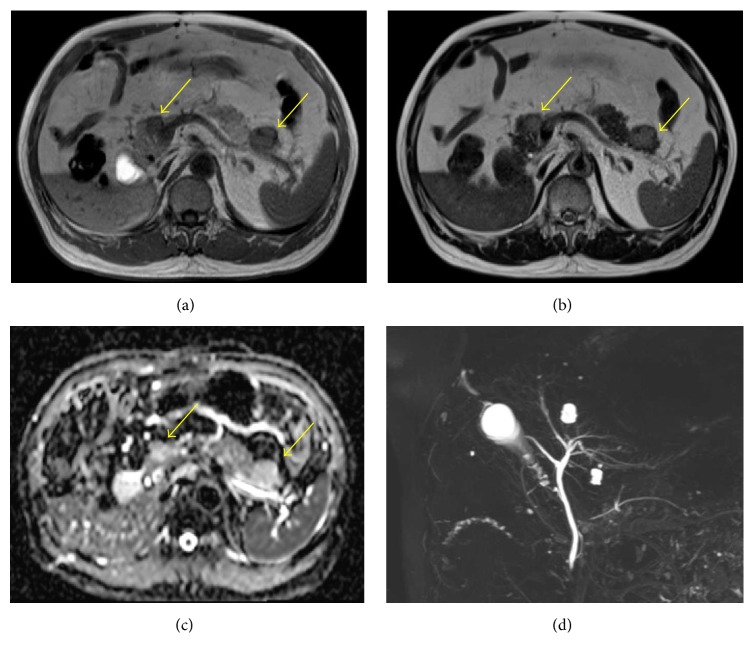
Magnetic resonance imaging sections of pancreas tumors*. Pancreatic tumors were shown as slightly high-intensity regions on T1-weighted imaging and as isointense regions on T2-weighted imaging (yellow arrow: (a), (b)). Diffusion-weighted imaging (DWI) revealed these masses as high-intensity regions (yellow arrow, (c)), and magnetic resonance cholangiopancreatography (MRCP) showed no stenosis and irregularity in the biliary duct and pancreatic duct (d).
